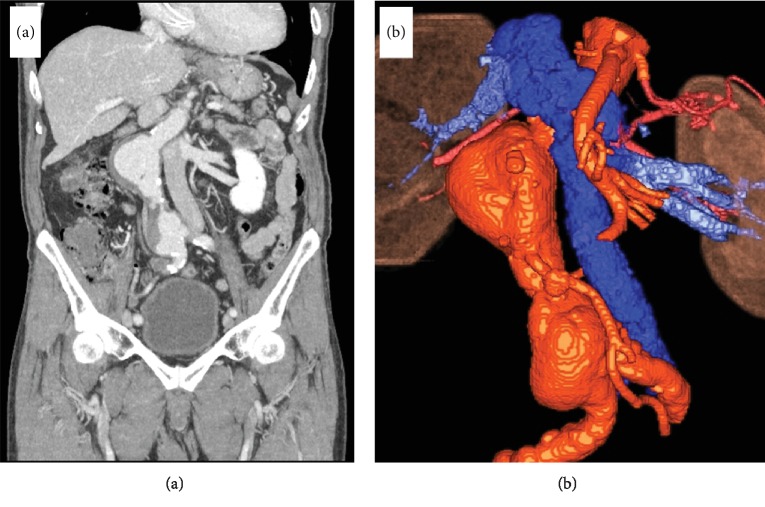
Infrarenal abdominal aortic aneurysm and left-sided inferior vena cava. (a) Computed tomography revealed that the inferior vena cava ran left to the abdominal aortic aneurysm (AAA). The AAA was 50 mm in diameter. (b) The left-sided inferior vena cava crossed the aortic neck anteriorly after receiving the left renal vein at the level of the bilateral renal arteries and ran right to the aorta in the normal position.
